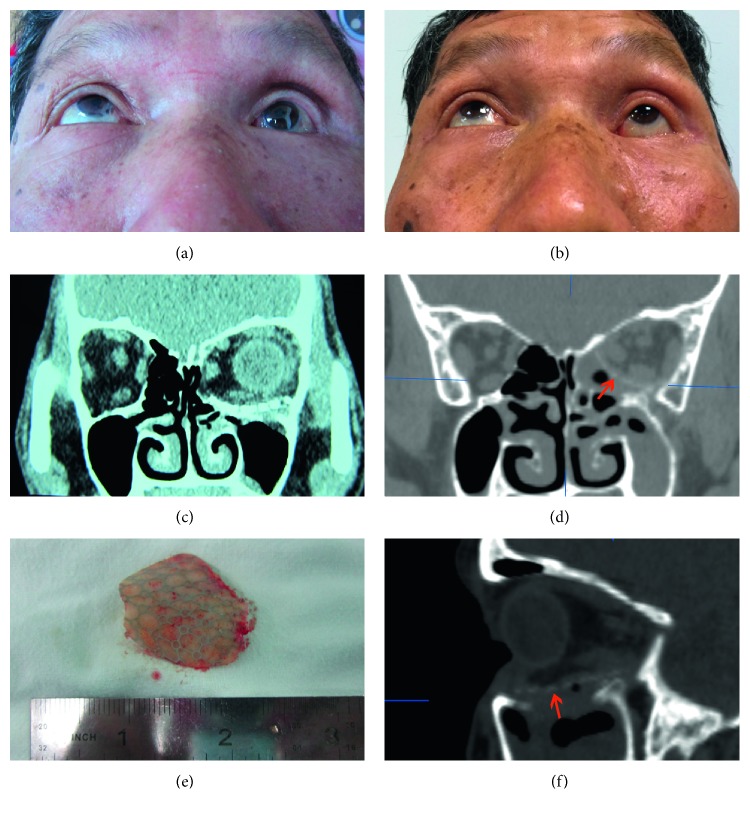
Case of a malpositioned Medpor Titan implant. (a) The left eye exhibited enophthalmos 5 months after primary surgery. (b) The enophthalmos was fully corrected over a 16 month follow-up. (c) The implant reconstructed only the wall of the orbital floor leaving the medial wall unrepaired. (e) The explanted Medpor Titan mesh. (d and f) A CT scan shows the newly positioned implant totally covering the orbital medial wall and floor defects (red arrow).
